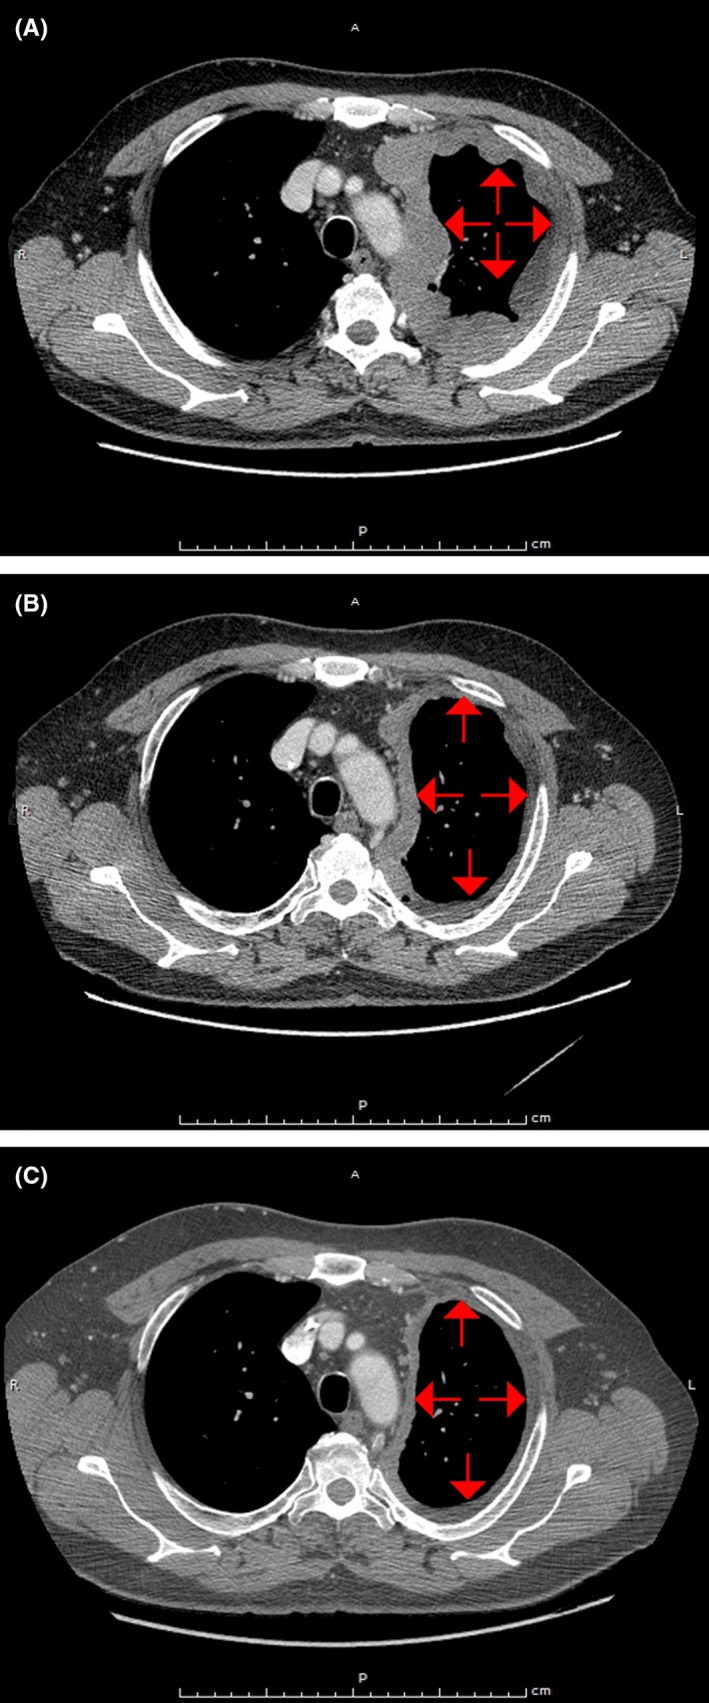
Disease reduction following nintedanib treatment. A, CT scan prior to nintedanib plus pemetrexed treatment. B, CT scan following 38 d of nintedanib treatment (plus pemetrexed plus cisplatin). C, CT scan following 129 d of nintedanib treatment (as maintenance therapy). Arrows show location of neoplasm. CT, computed tomography; d, days
